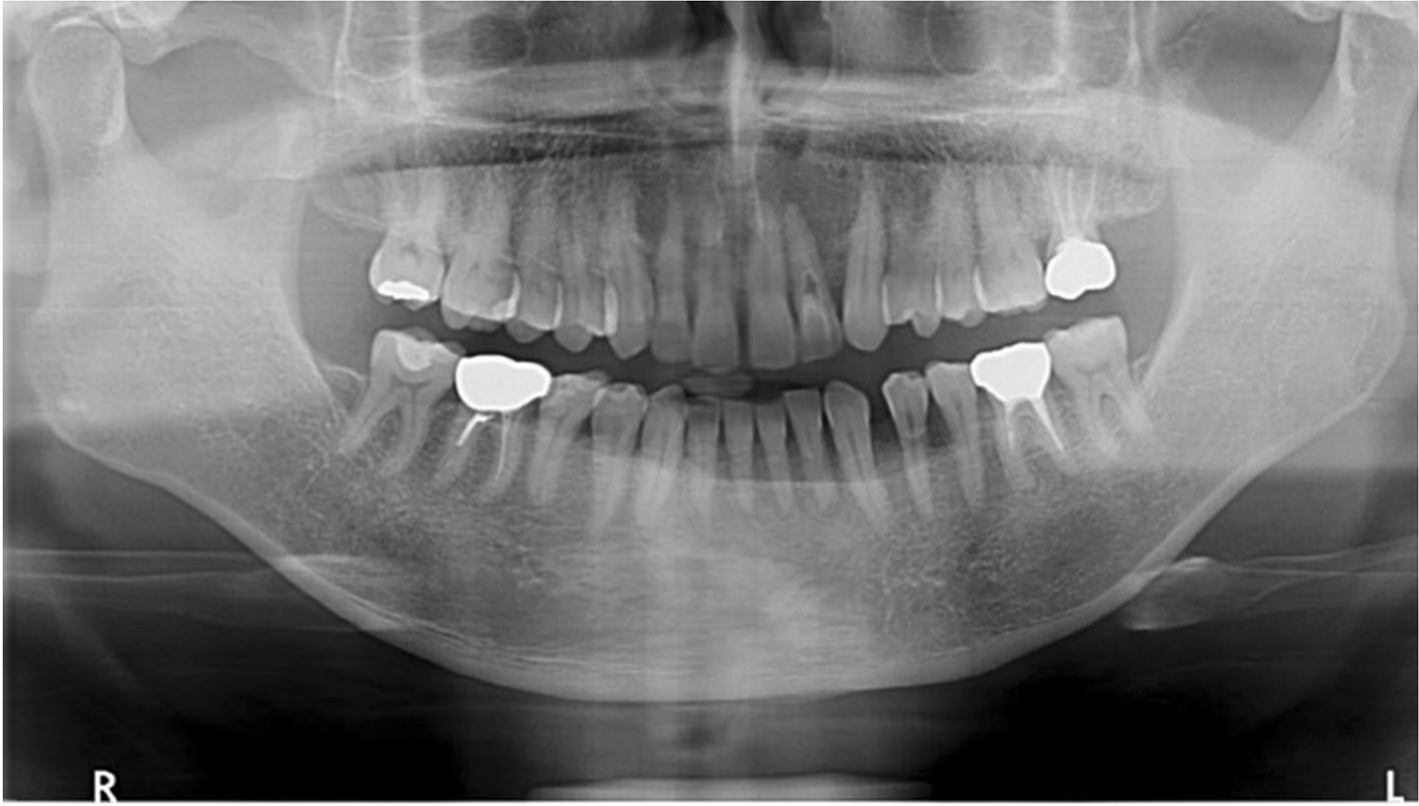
Dental panoramic radiograph or pantomograph.
